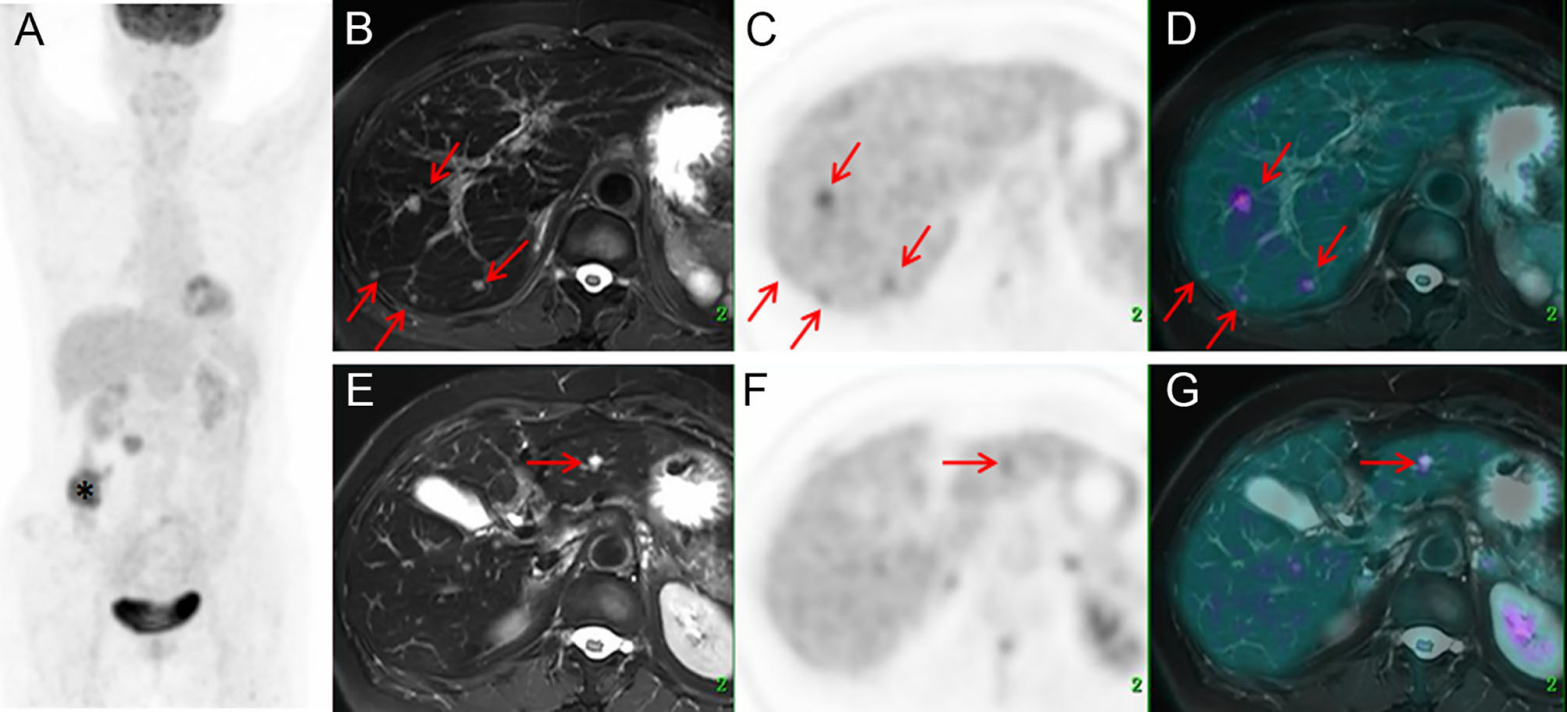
Images of a 64-year-old female with colonic mucinous adenocarcinoma. The MIP (A) of PET/CT showed a primary lesion at the ascending colon (*) with no [^18^F]FDG foci on the liver. Delayed T2WI, PET, and PET/MR-merged images showed multiple metastases (red arrow) with hyperintensity and hypermetabolism on the right lobe (B–D) and left lobe (E–G).
